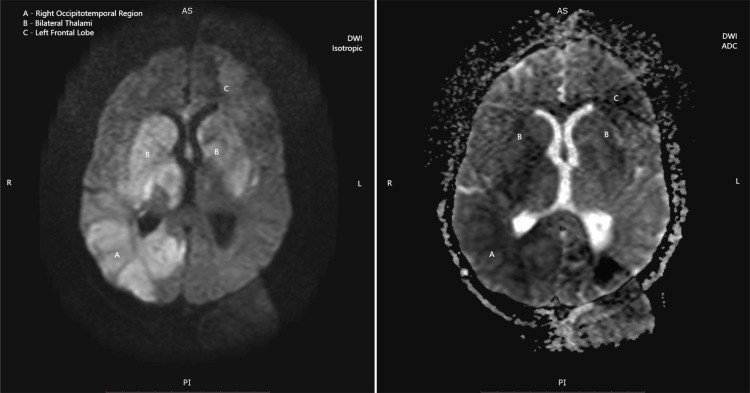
Diffusion-weighted MRI (DWI isotropic, left) and corresponding ADC map (right) highlighting multifocal areas of diffusion restriction ADC, Apparent diffusion coefficient; DWI, Diffusion-weighted imaging; MRI, Magnetic resonance imaging
